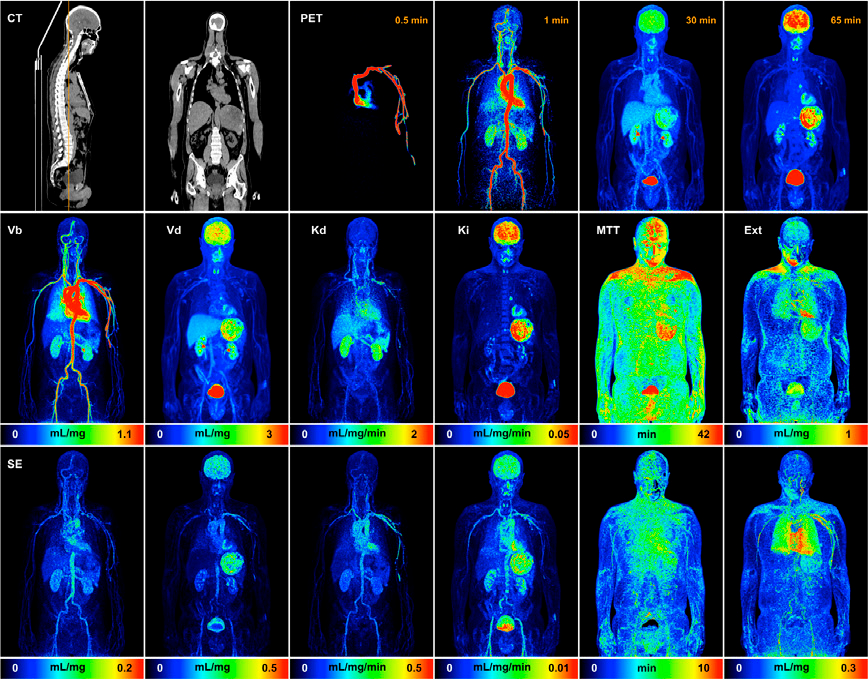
MIP maps of NPRM kinetic parameters and associated SE. SEs are based on SD of MIP results for each of 25 bootstrap replications. Top row shows CT images for selected cross sections through volume and PET MIP maps at indicated times. *K* = 9 basis elements were determined for data by NPRM methodology. MTT = mean transit time; Ext = extraction fraction.
